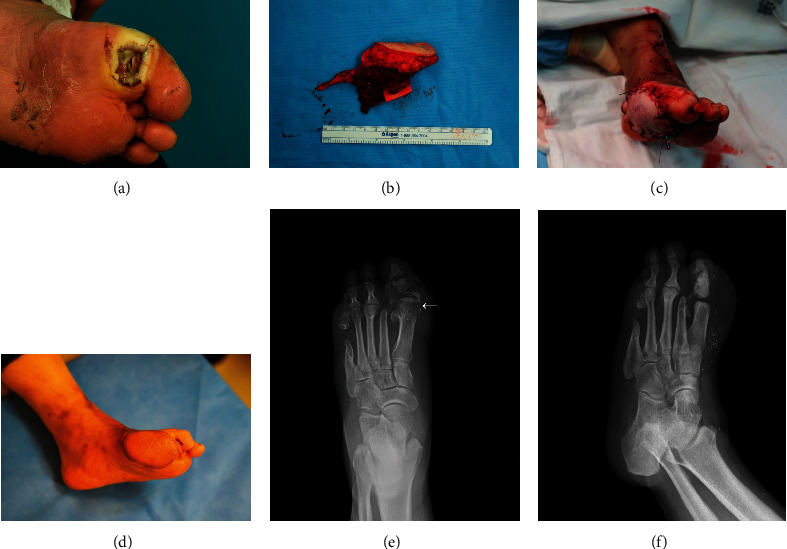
(a) A 50-year-old woman with an recurrent diabetic foot ulcer involving the big toe. (b) Harvested free lateral arm osteomyocutaneous flap. (c) Immediate postoperative image. (d) Postoperative 13 months. (e) Preoperative X-ray image shows osteomyelitis lesion from the proximal phalanx of the left first toe (white arrows). (f) Postoperative X-ray image shows successful bone replacement by filling the first proximal phalanx with the bony portion of osteomyocutaneous flap.
